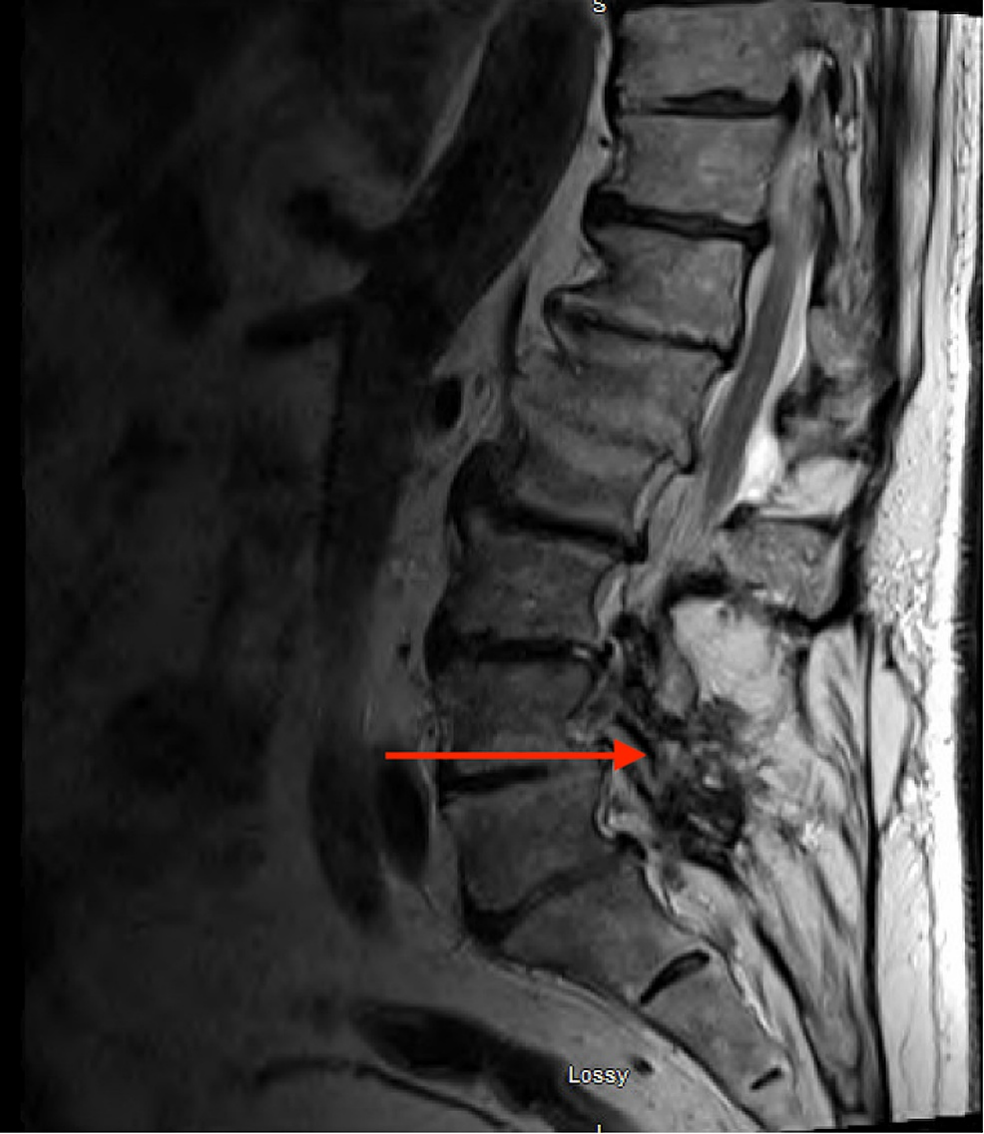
MRI spine without contrast showed disc bulging with development of fluid within the disc space and right paraspinous inflammation without the abscess MRI, magnetic resonance imaging
